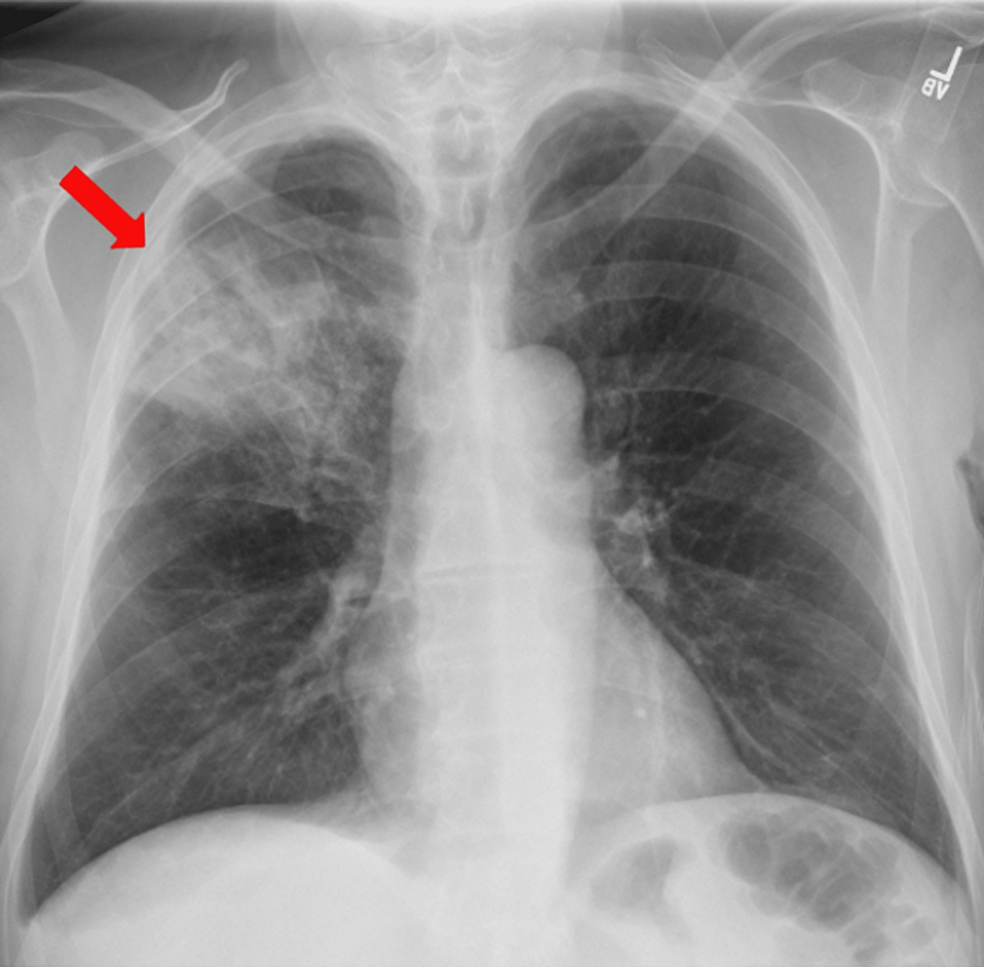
Chest radiograph from the day of admission demonstrating a right upper lobe cavitary lesion (red arrow).
